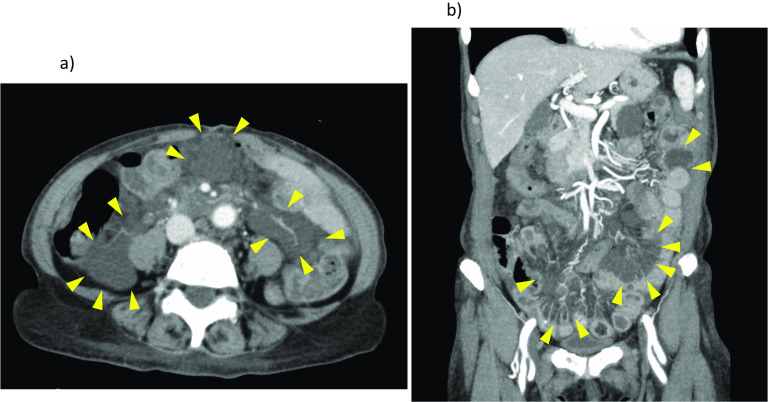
Enhanced abdominal computed tomography: Multiple low-density areas of mesentery in a axial and b coronal views, devoid of contrast effect (arrowhead)
